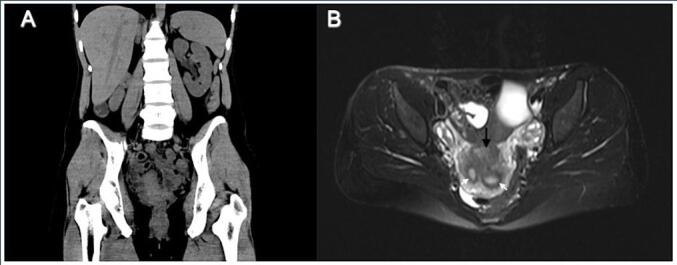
Imaging findings demonstrating anomalous anatomy. A. Coronal CT demonstrating evidence of absent right kidneyB. Axial MRI demonstrating vaginal mass (black arrow) and bicornuate uterus (white arrow noting each uterine horn).
